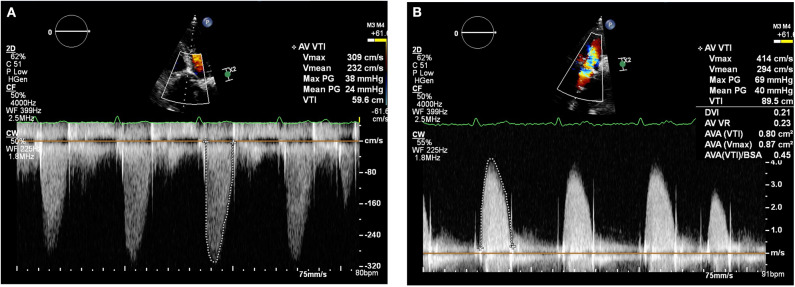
Transthoracic echocardiogram (January 2018): Continuous wave doppler in the apical 5 chamber view (A) and right parasternal long axis view (B) demonstrating a maximum transaortic velocity of 4.1 m/s.
